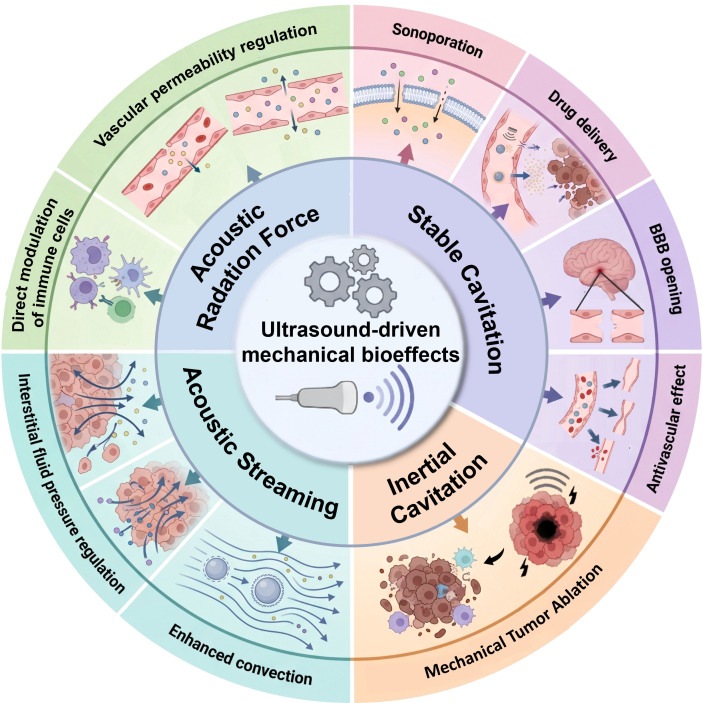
Ultrasound-driven mechanical bioeffects in tumor therapy.
